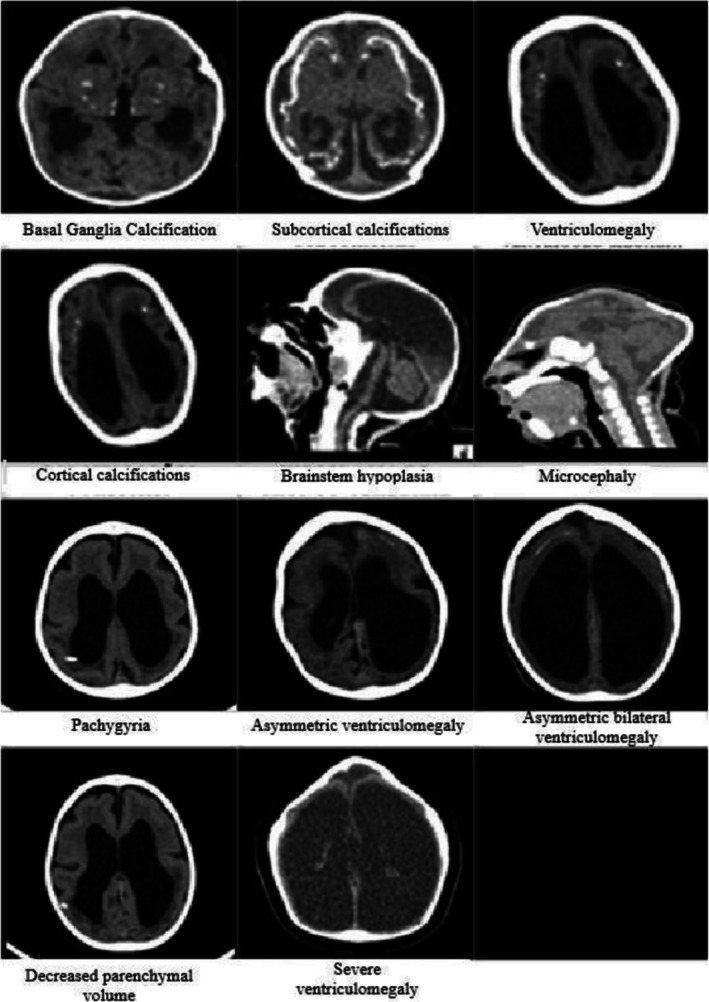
Alteration findings in head computed tomography of children with congenital Zika virus syndrome, treated at the Sarah Neurorehabilitation Center. Fortaleza, CE, Brazil, 2019.
